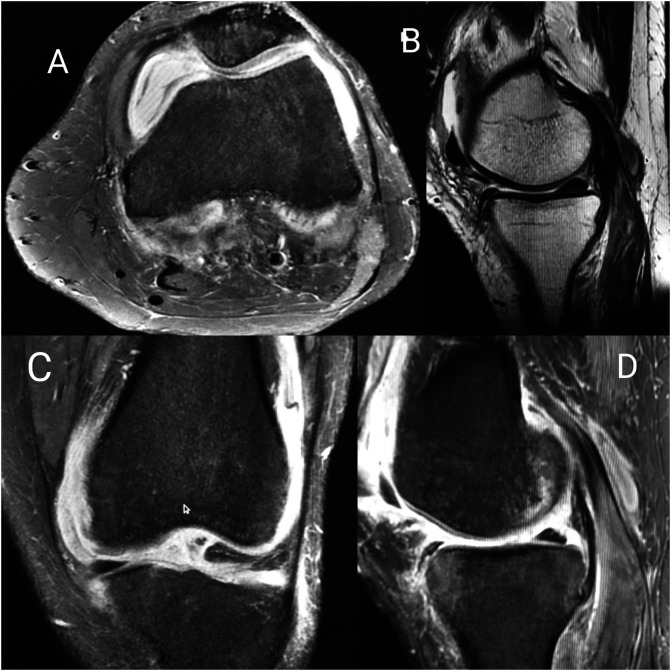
Figure showing MRI sections of a patient with inflammatory synovitis (A – axial PDFS, B – sagittal T2, C – coronal PDFS, sagittal PDFS showing hyperintensity in subarticular marrow of the tibia and femur with post-contrast enhancement and moderate effusion with diffuse enhancing synovial thickening).
